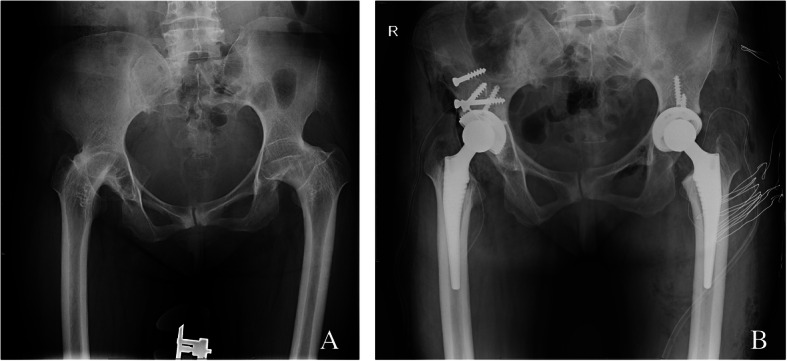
a Patient 1, Preoperative x-rays with double hip fusion. b The full-screw screws were nailed into the fracture ends, and the screw caps were replaced with steel wires in the form of cross-shaped bundling and compression
